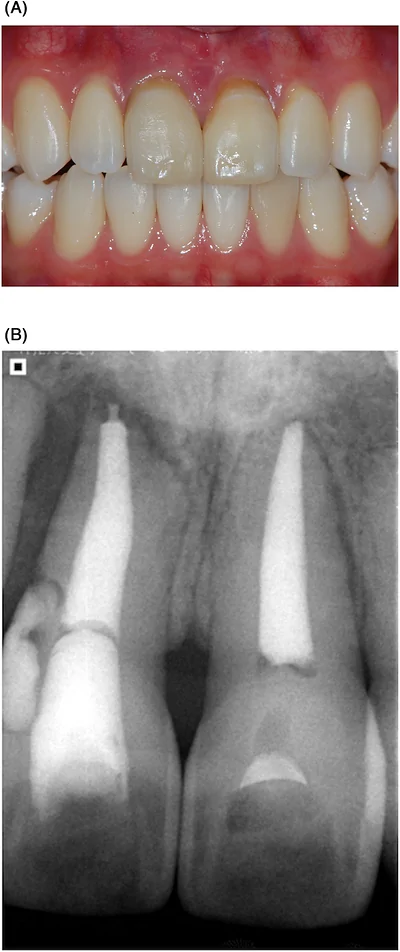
(A) Clinical image after 16 years. (B) Radiograph image after 16 years.
